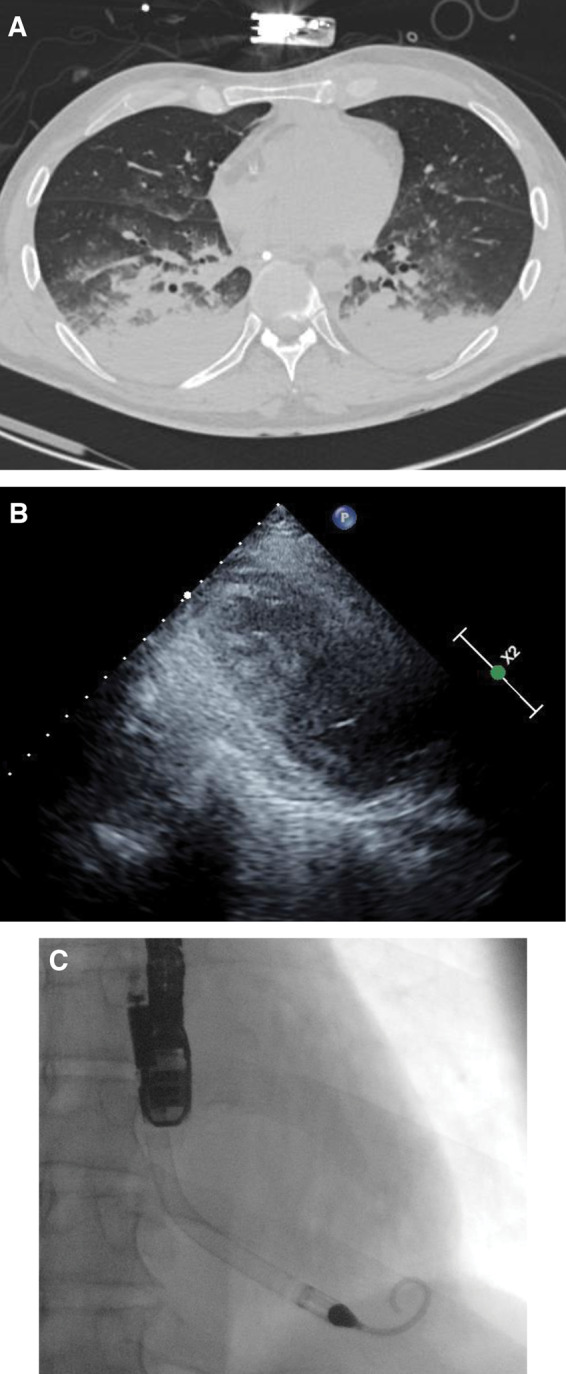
(*A*) CT chest consistent with interstitial lung infection. (*B*) Trans-thoracic echocardiograph with spontaneous echo contrast, on peripheral VA ECMO, prior to Impella implant. (*C*) Trans-oesophageal confirmation of Impella position.
